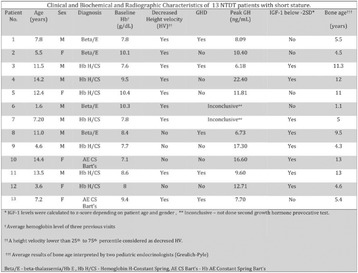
Clinical and biochemical and radiographic characteristics of 13 NTDT patients with cohort stature
